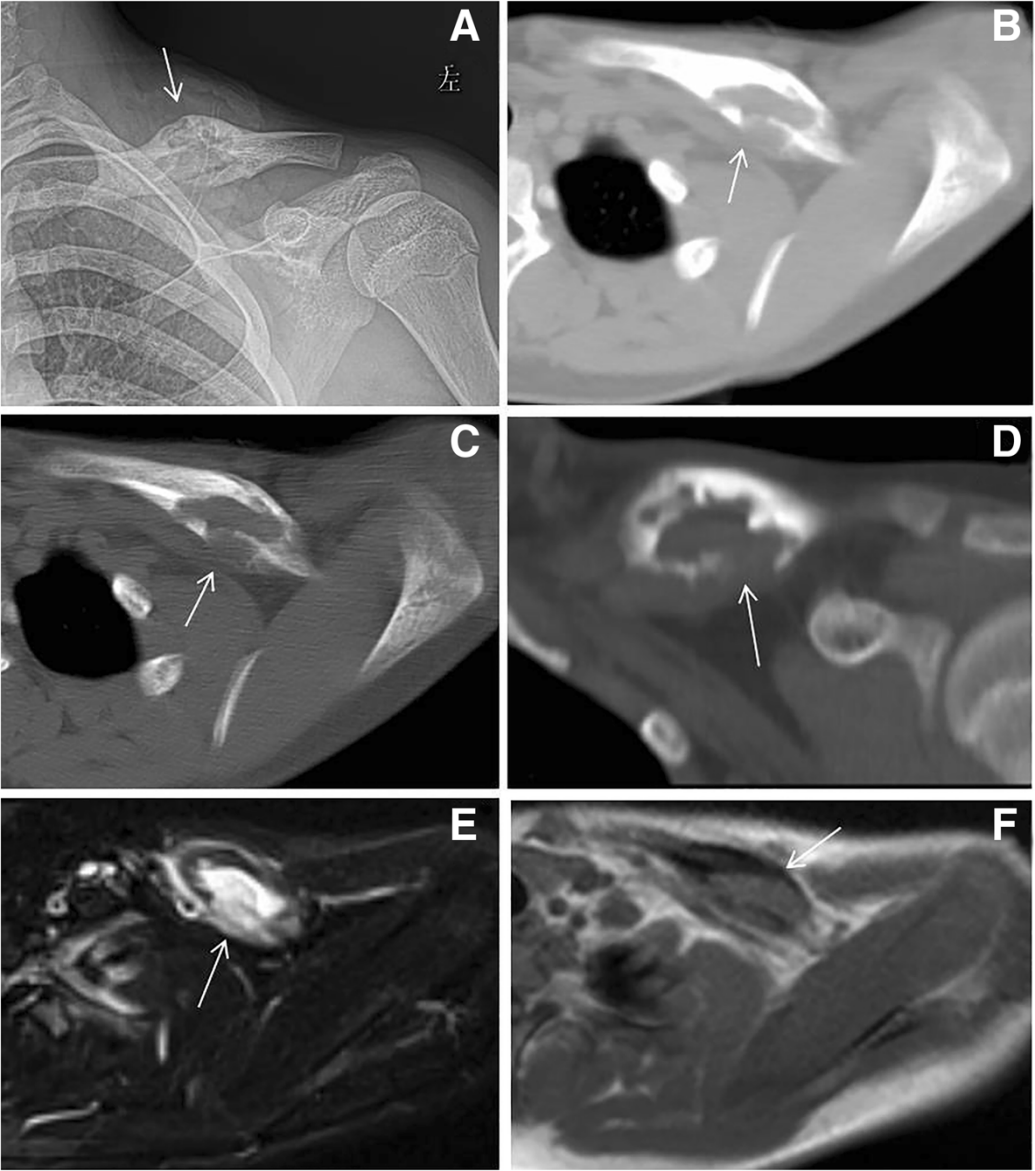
Fourteen-year-old male presenting with left clavicular lesion. a Expansive bone destruction on the left clavicle (arrow). b There was a destructive mass with a prominent soft tissue component (arrow) demonstrated on axial non-contrast computed tomography (CT) in soft tissue window. c, d Axial and coronal CT in bone window showed hyperosteogeny and sclerosis (arrow). e The mass had components that was hyperintense (arrow) to region on axial STIR-weighted magnetic resonance imaging (MRI). f Axial MRI T1 sequence revealed soft tissue mass (arrow)
